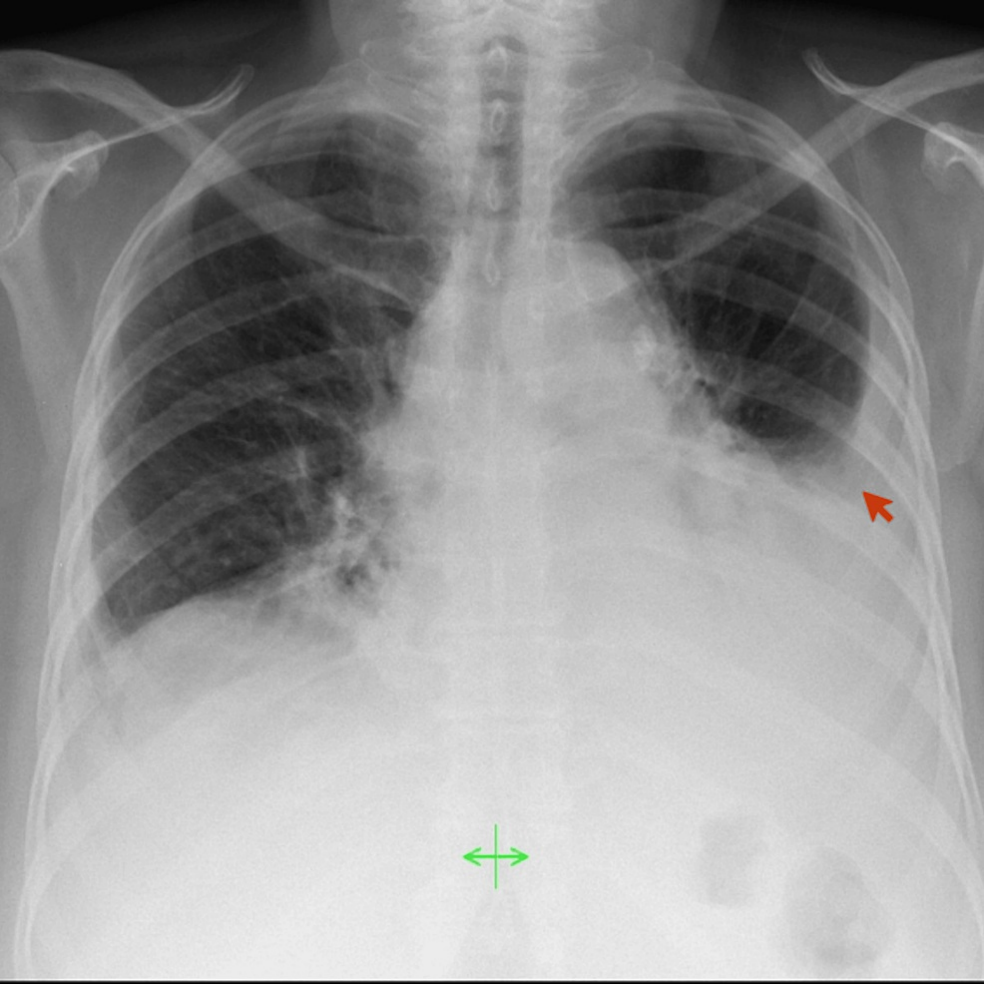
Chest X-ray showing bilateral pleural effusion but more evident on the left side, as indicated by the red arrow
